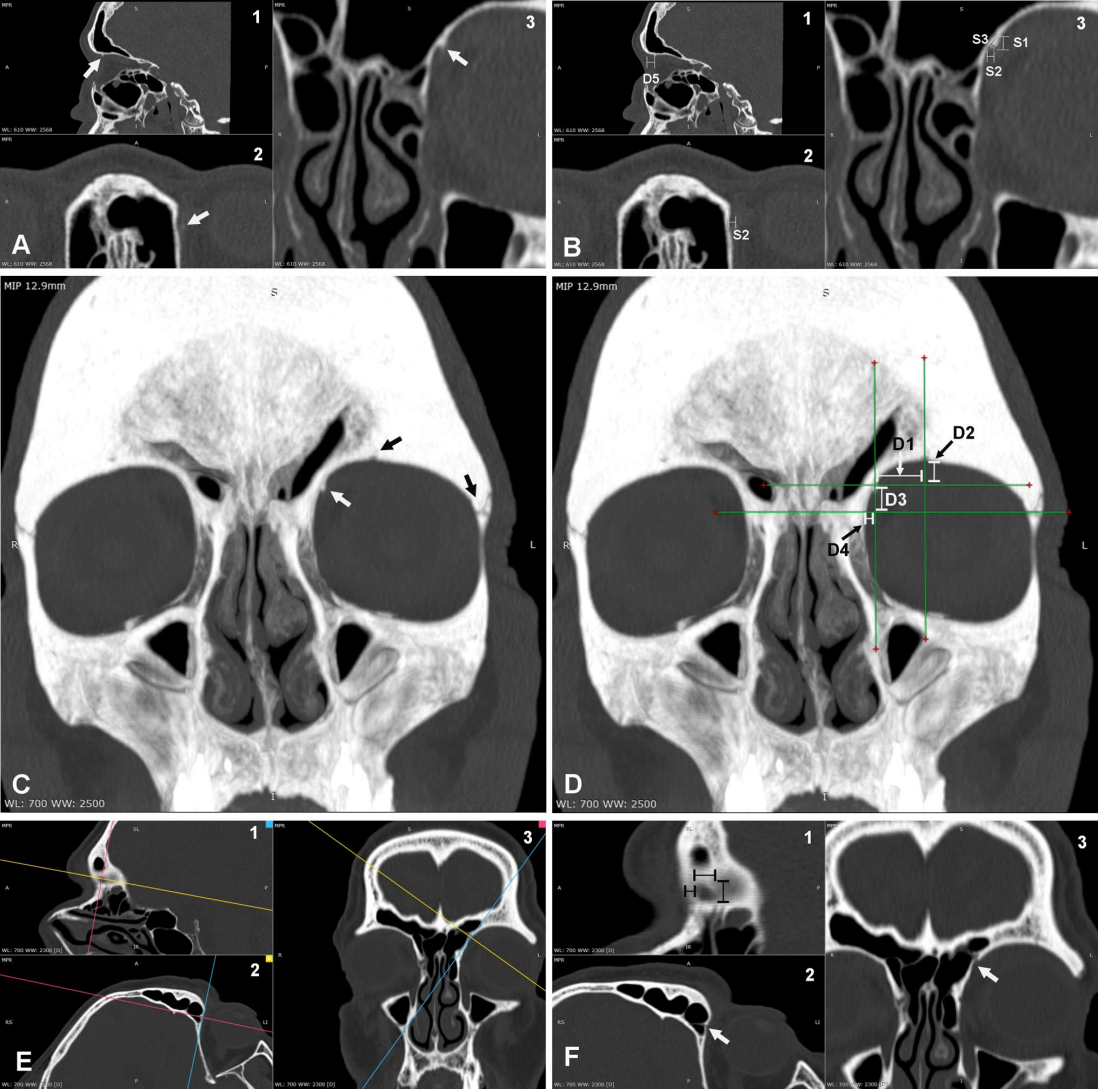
Morphometric methodology employed in this study for the trochlear spine and fovea. Procedures followed for their identification and measures. A Identification of the trochlear spine (arrows) in a left orbit by CT on the sagittal (A1), axial (A2) and frontal plane (A3). B Measurements taken on the planes shown in B: S1 = vertical projection of the spine tip from the orbital roof as seen in B3; S2 = axial projection of the spine tip from the medial wall as seen in B2 and B3; S3 = distance measured along a perpendicular line between the spine tip and the orbital wall as seen in B3; D5 = distance of the spine from the orbital rim as seen in B1. C, D Measurements taken according to Aglianò’s references (2018). In C it is shown the maximum intensity projection (MIP) tool that was applied to visualize a frontal slice of the skull as thick as 12.9 mm (in the present example) in order to observe on the same image both the trochlear spine (white arrow) and the bony landmarks, supraorbital notch/foramen and frontozygomatic suture (black arrows). E, F TF identification by CT on a left orbit. Once a small indentation adjacent to the superior oblique tendon reflection is observed on standard projections (a candidate for TF identification), the sagittal plane is reoriented to be tangent the surface of the orbital wall so that the TF can be seen as a small depression completely surrounded by a bony rim (E1). E2 and E3 show the sagittal plane tangent the TF whereas F shows the enlarged images of the planes seen in E. White arrows in F2 and F3 point to the trochlear fovea. In F3 the fovea is seen as a small pit surrounded by a bony rim. Diameters and distance from the orbital rim are taken as shown
